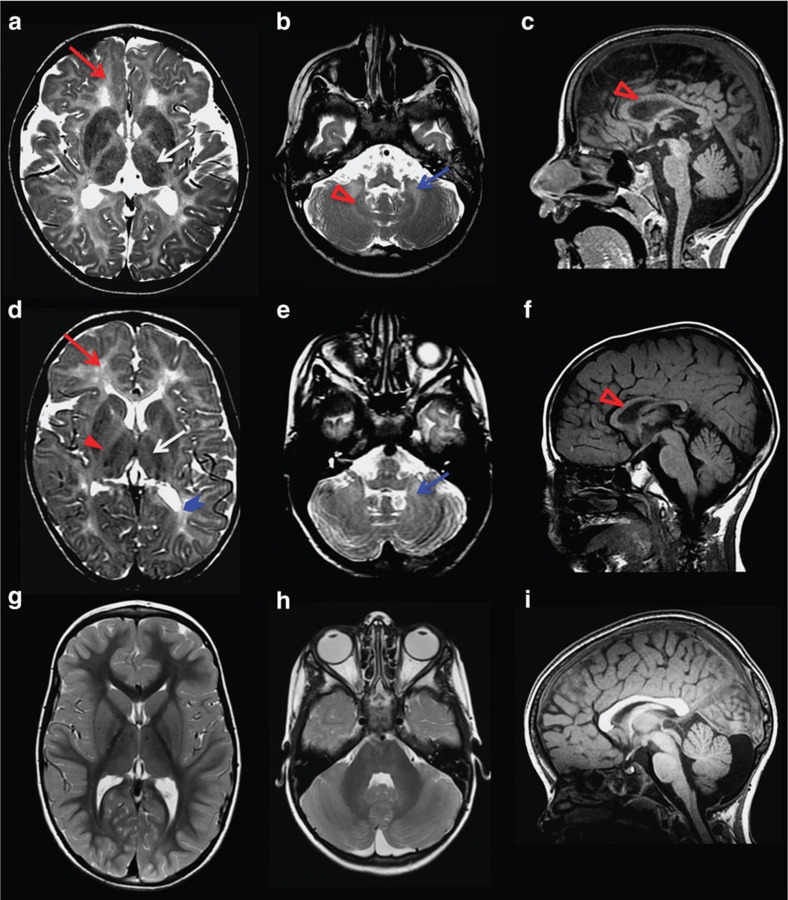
MRI characteristics of POLR3-related leukodystrophy caused by *POLR1C* mutations. Axial T2-weighted (a,b,d,e,g,h) and sagittal T1-weighted (c,f,i) images of case 1 aged 6 years (a–c) and case 2 aged 4.5 years (d–f) compared with a healthy control aged 4 years (g–i). Diffuse hyperintense signal of the supratentorial (red arrow, a,d) and cerebellar (blue arrow, b,e) white matter is visible on the T2-weighted images, indicating hypomyelination. There is no cerebellar atrophy. As typical for POLR3-related leukodystrophy, the ventrolateral thalamus (white arrow, a,d), the optic radiation (thick arrowhead blue, d) and the dentate nucleus (open red arrowhead, b) show a relative hypointense signal on the T2-weighted images resulting in an easily visible dentate nucleus (b) as compared with the control (h) as well as a small dot in the posterior limb of the internal capsule (red arrowhead, d). The corpus callosum is slightly thinned in case 1 and thinned in case 2 (open red arrowhead, c,f).
